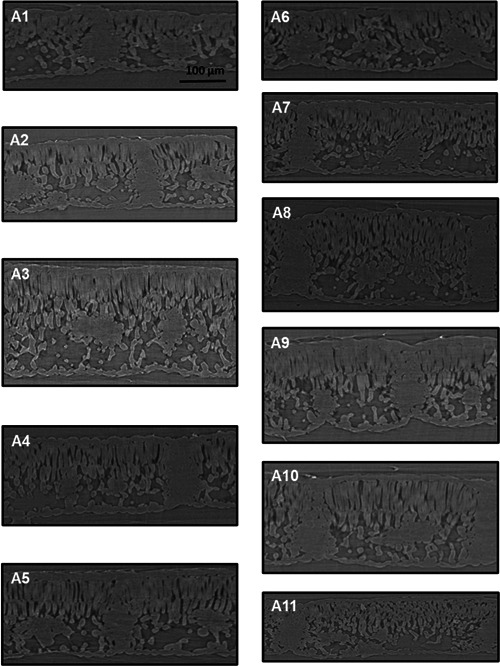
Leaf cross sections from representative scans for 11 *J. regia* accessions under well‐watered condition obtained using X‐ray microcomputed tomography.
